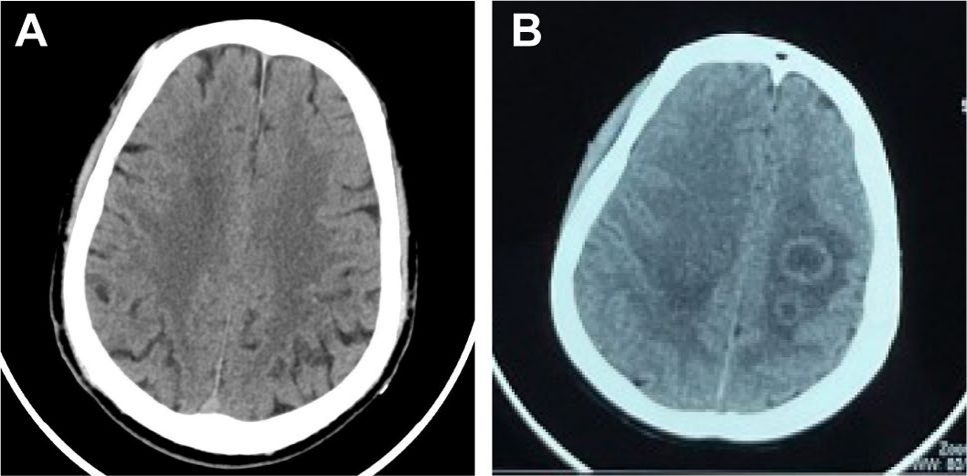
Head CT at different stages. (A) Head CT showed no apparent abnormalities. (B) Head CT scan (13 days after the accident) showed multiple rings of slightly high-density shadows with slightly low-density shadows around
